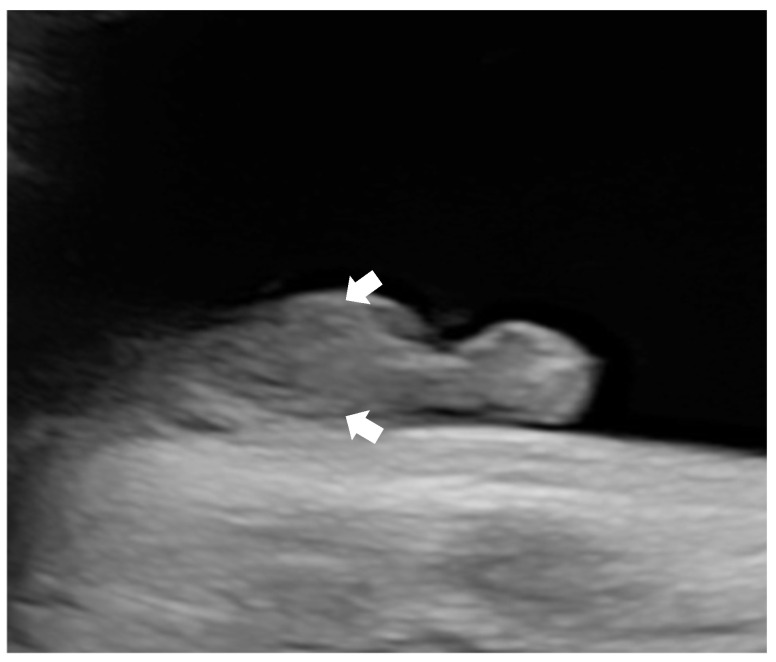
Ultrasonographic image of bilateral crytorchidism (arrows).
